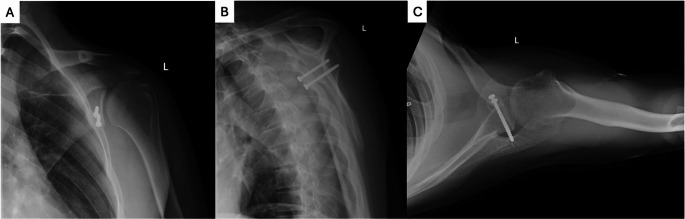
Post-operative Radiograph Views of Latarjet Patient: (a) AP Oblique View; (b) Scapular Y View; and (c) Axillary Lateral View
